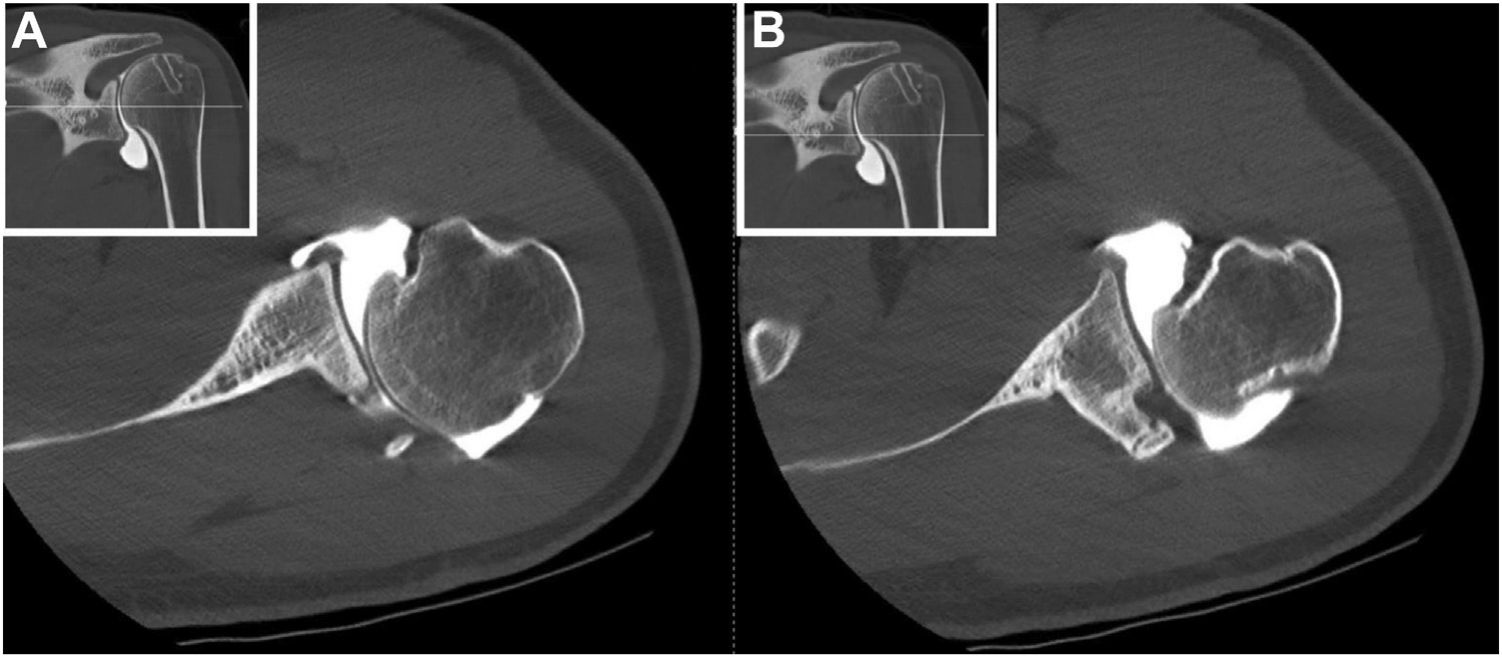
(A) CT arthrogram of the left shoulder demonstrating severe posterior subluxation of the humeral head, with absence of the superior, middle, and inferior glenohumeral ligaments. (B) Lucencies within the humeral head demonstrate removal of hardware from prior surgery. Presence of failed posterior iliac crest bone block graft, with chronic nonunion. *CT*, computed tomography.
